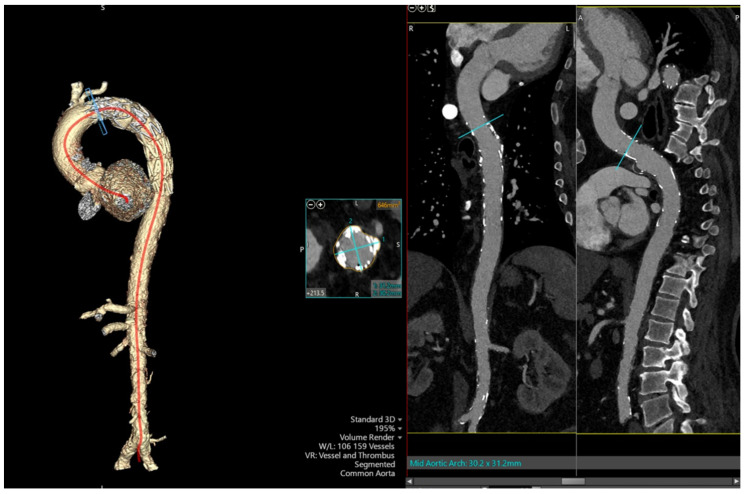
Cross-section of CT imaging with 3D reconstruction of the arch of the aorta and the descending aorta with a stent in situ (left), and coronal view of the stent graft from the arch of the aorta into the proximal descending aorta (right).
